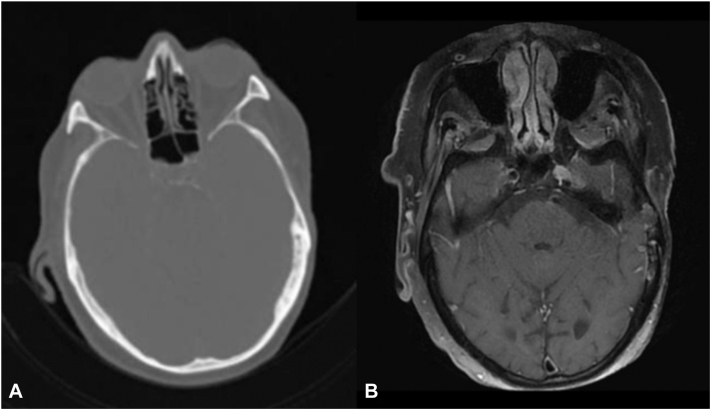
A, PET/CT demonstrating no evidence of local or bony invasion. Focal avidity at the left EAC (standardized uptake value 3.6). B, MRI head and neck: No abnormal calvarial bone mineralization, no soft tissue mass or mass-like enhancement, stable minimal soft tissue thickening within the left EAC, suspected to be consistent with chronic inflammatory change. *CT*, Computed tomography; *EAC*, external auditory canal; *MRI*, magnetic resonance imaging; *PET*, positron emission tomography.
